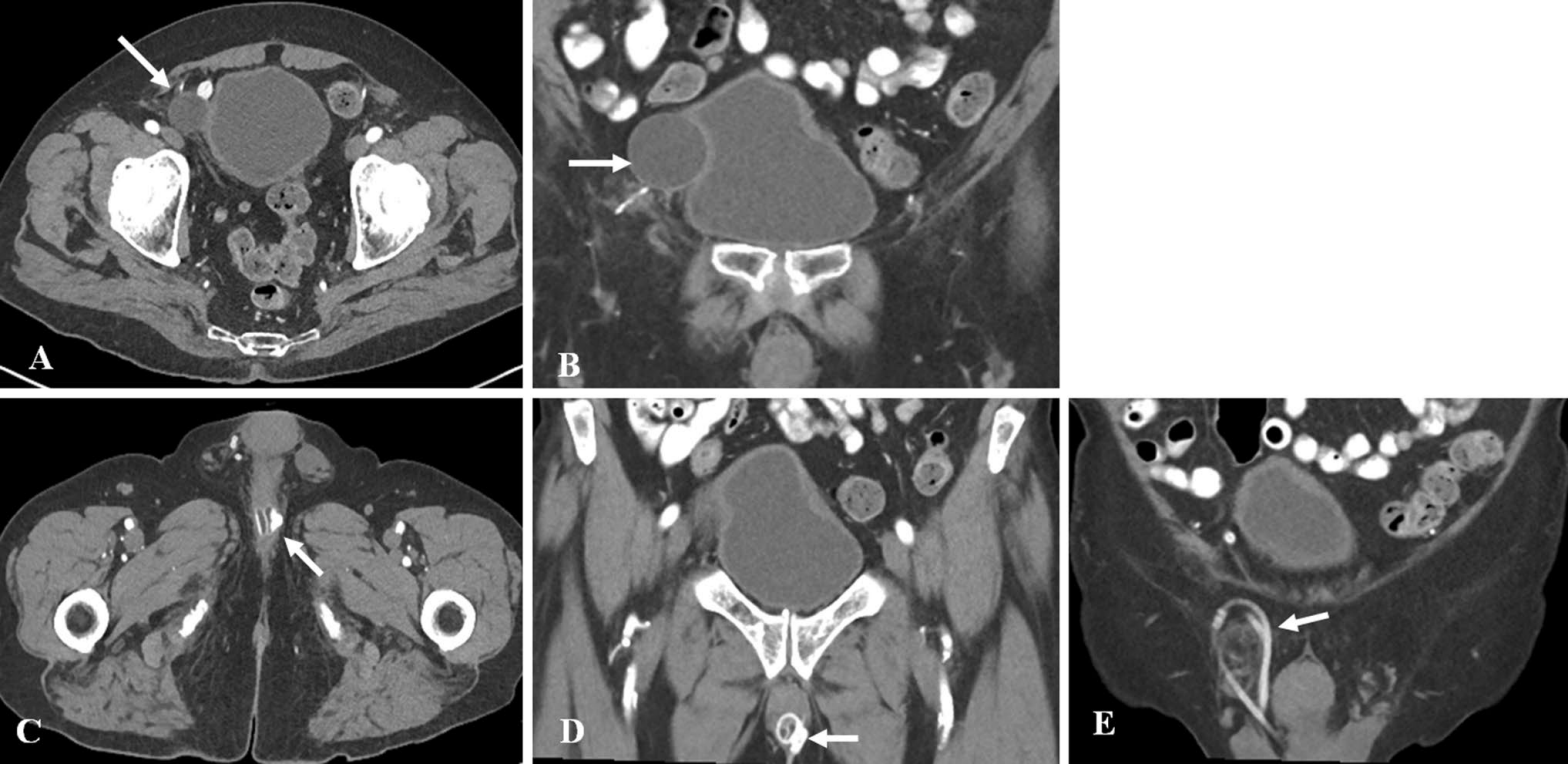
Appearance of uncomplicated AUS on CT in a 68-year-old male with florid incontinence after radical prostatectomy. a Axial contrast enhanced CT shows the reservoir (arrow) in the right pelvis in the space of Retzius. The reservoir indents the right side of the bladder, seen better on the coronal image (1b). It is important not to misdiagnose the reservoir as a cystic lesion in the pelvis. b Coronal view demonstrates the saline filled AUS reservoir (arrow) indenting the bladder. c, d Axial and coronal views more inferiorly shows the sphincter cuff (arrows) around the bulbar urethra. e Coronal view shows tubing related to the AUS coiled in right hemiscrotum (arrow)
